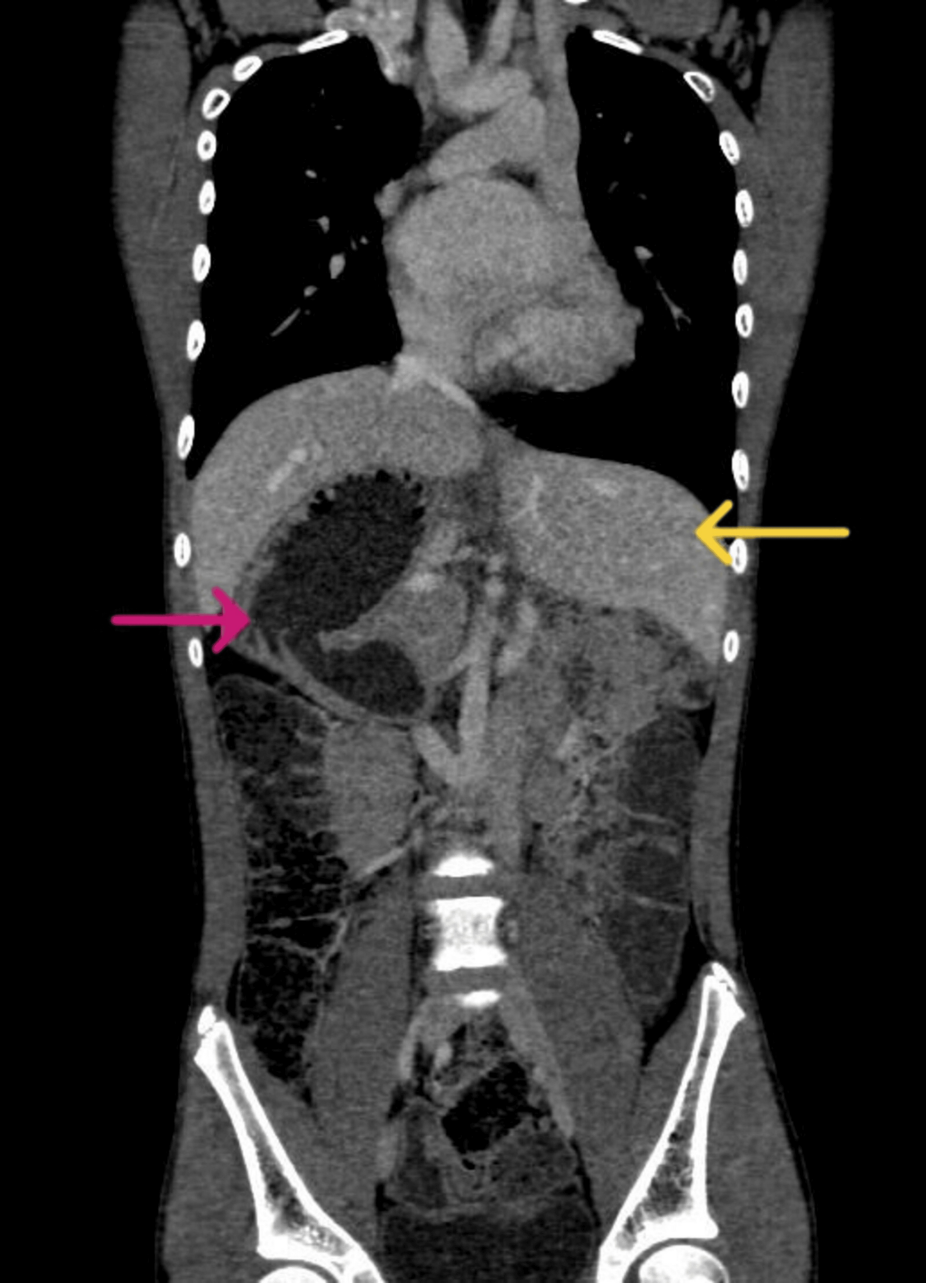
Computed tomography of the abdomen (coronal view) showing situs inversus with liver in the left hypochondrium (yellow arrow) and right-sided stomach (pink arrow).
